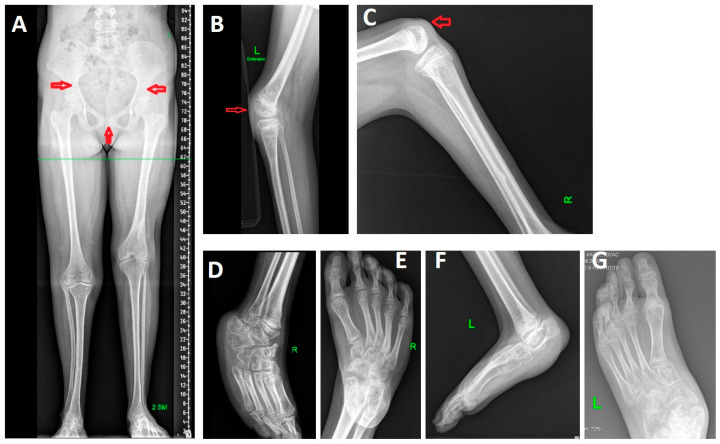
Radiographs of the proband’s lower limbs. (A) Panoramic anteroposterior radiograph of the lower limbs of a 17-year-old female patient. A shortening of the left lower limb by 2 cm is observed (4 cm in the right tibia and 6 cm in the left femur). The iliac bones exhibit an atypical shape, and the pubic symphysis is widened (indicated by red arrows). (B,C) Lateral radiographs of the knees of a 12-year-old female patient. Flexion contractures are present, measuring 70° on the right and 35° on the left. Bilateral patellar aplasia is evident (indicated by red arrows). (D–G) Anteroposterior and lateral radiographs of the feet of an 11-year-old female patient. The right foot demonstrates an equinovarus deformity (D,E), while the left foot shows an equino–plano–valgus deformity (F,G).
